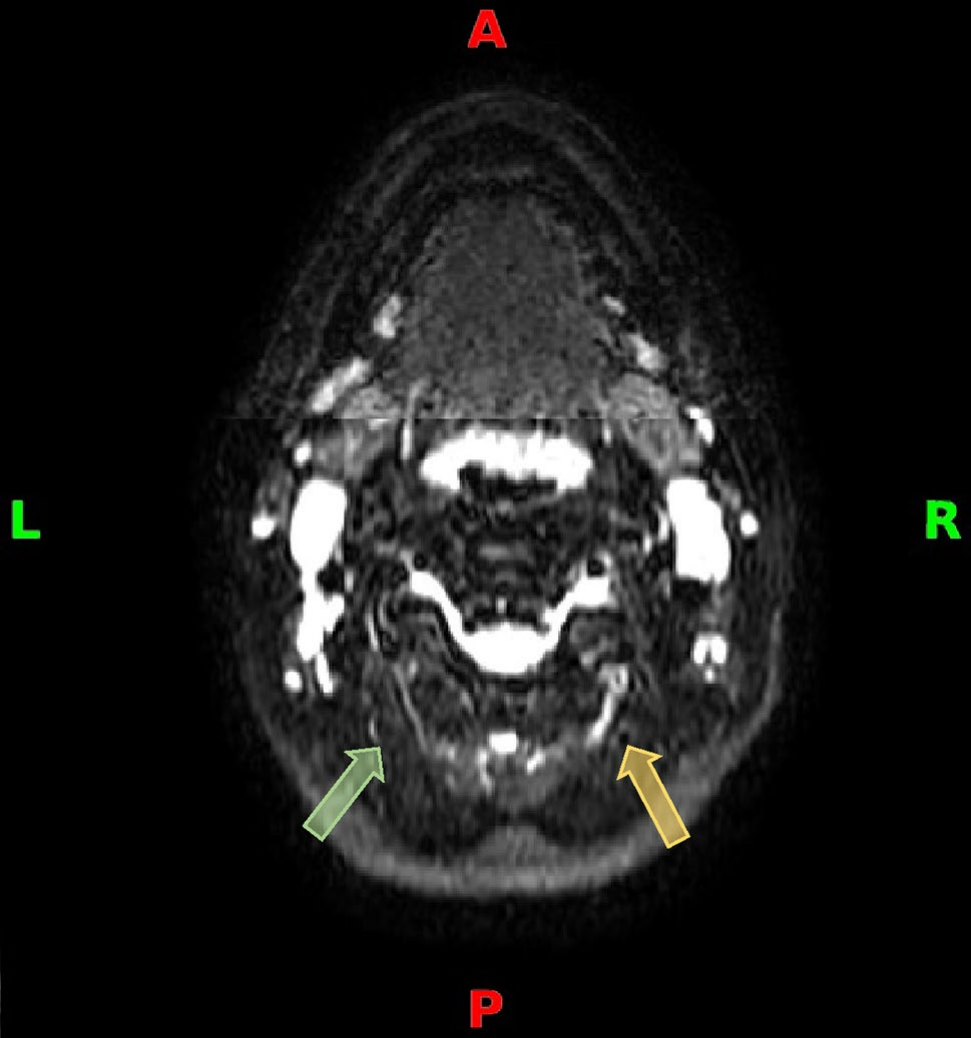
Transverse NerveView MRI tracking the right GON (yellow arrow) with high intensity signal as compared to the left GON (green arrow).
